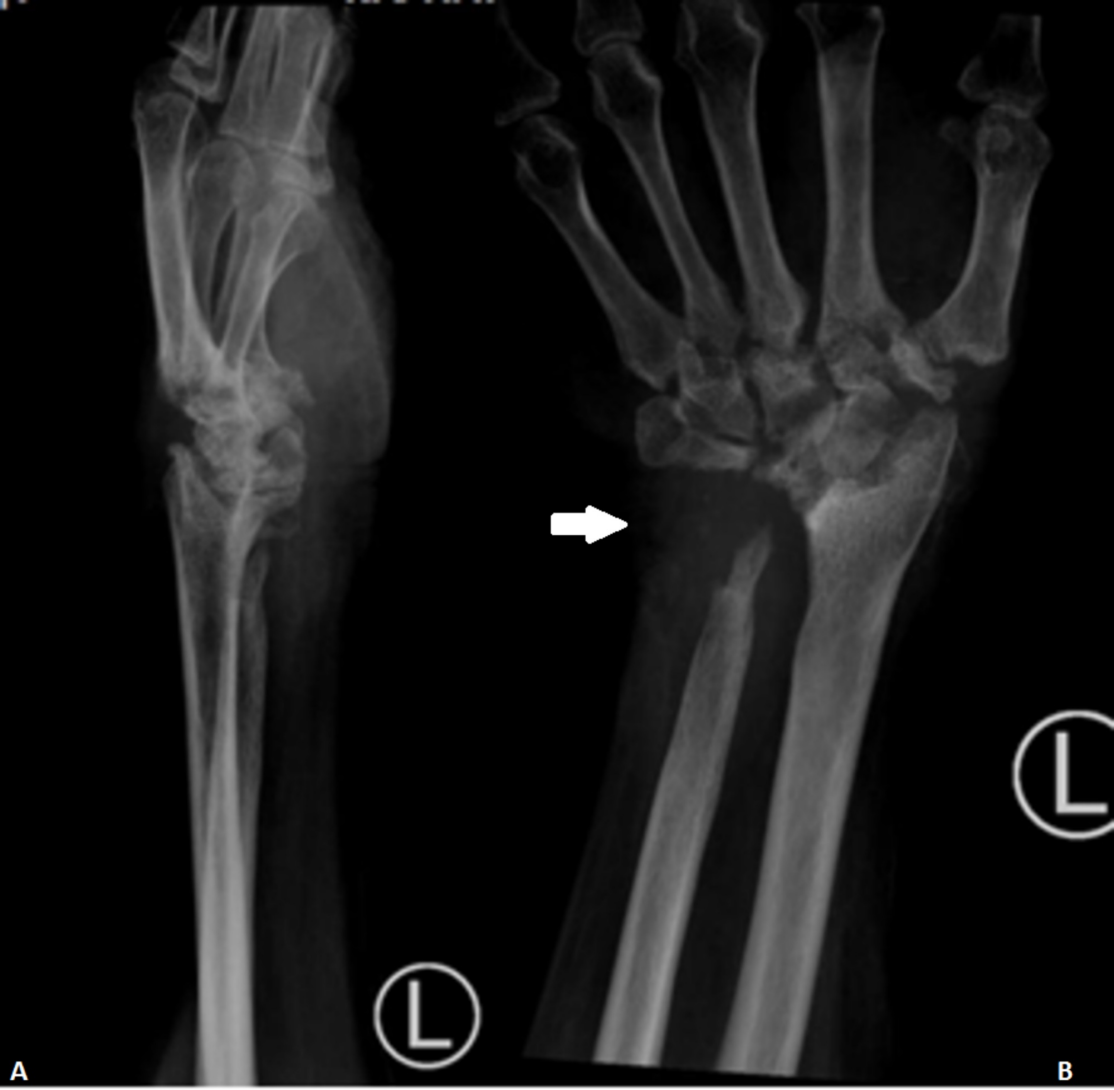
X-ray film of the left wrist after one year of antituberculous therapy. Based on Figure 4B, there is erosion and a lytic lesion around the bony skeleton at the wrist. Mild reduction of the bony density is seen at the carpals and metacarpals. A : lateral view; B : antero-posterior view
